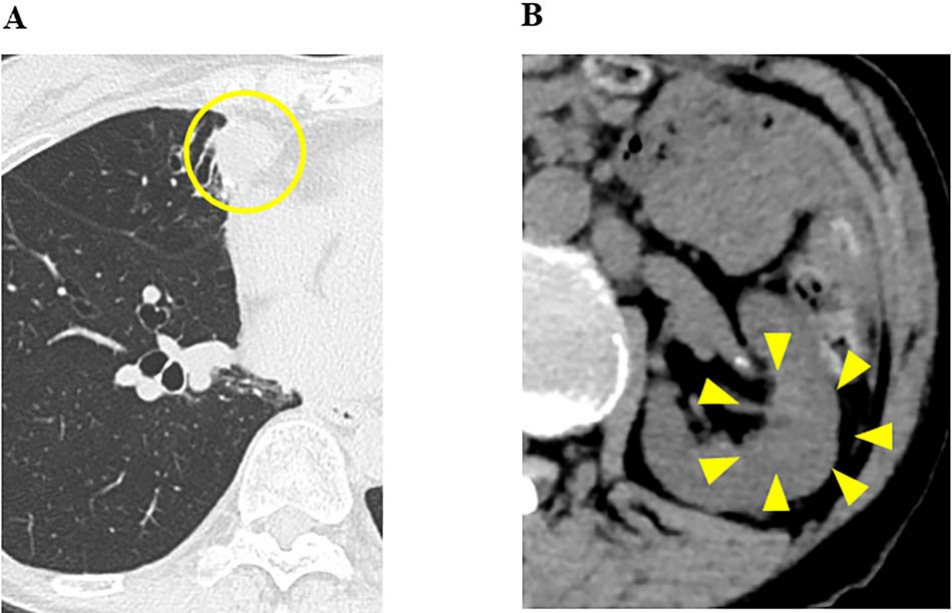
CT images of the chest and abdomen 10 months after the start of the administration of lenvatinib, and (A) pulmonary metastases decreased in size; (B) the size of the renal tumor decreased and the tumor plug disappeared.
